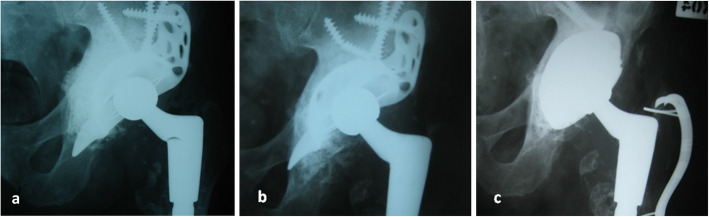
a Postoperative radiograph after reconstruction of acetabular defects with tricalcium phosphate/hydroxyapatite bone graft substitute and antiprotrusio cage in 77 year-old patient. A minor medial displacement of BoneSave particles is presented, caused by intraoperative crack during its compression. b Cage migration after 12 months, graft is compressed and partially incorporated. c After re-revision with oblong cup
